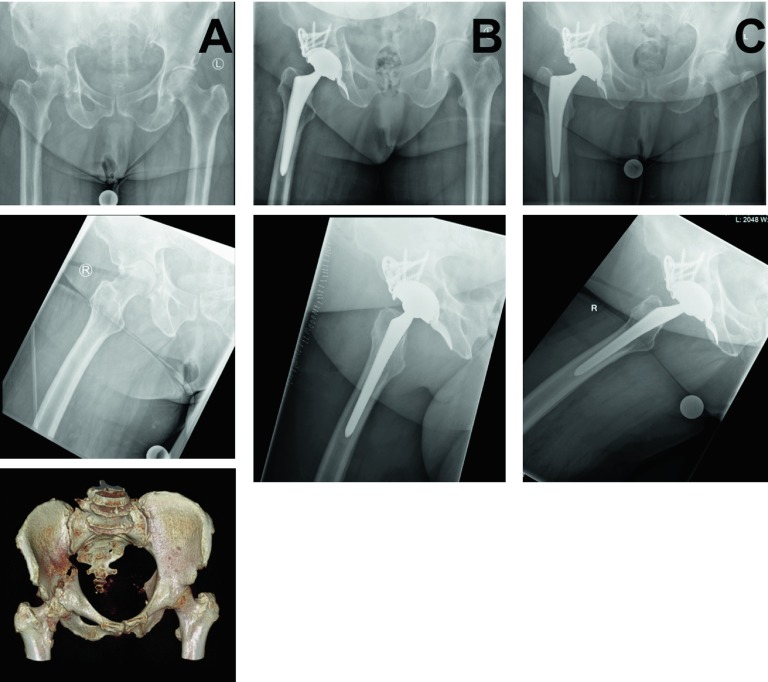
Fracture of the right acetabulum of a 67-year-old female. The patient reported to the emergency room four weeks delayed, upon admission impaction of the femoral head and the pattern of a two-column fracture was visible (column A). Significant comorbidities were missing, the individualized treatment plan was defined as acute total hip replacement (THR) with application of the Burch-Schneider reinforcement ring. Follow-up X-rays at two weeks (column B) and at three months (column C) are shown.
